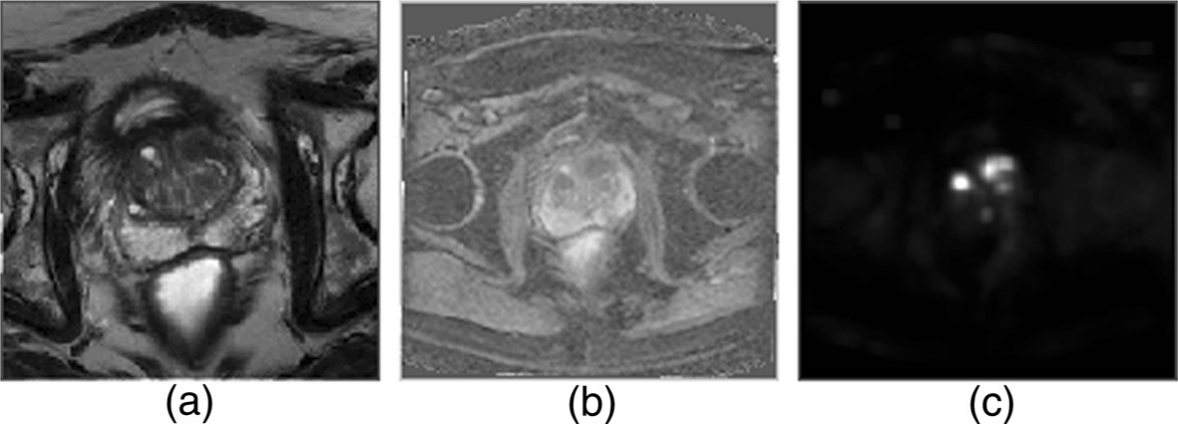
Example case 3. Tumors stand out well on CDI and poorly on T2-weighted imaging in patient with prostate cancer. a), T2-weighted imaging shows mild decrease in signal towards left side of transition zone. b), ADC map shows increased contrast around both sides of transition zone. c), CDI shows very high signal intensity corresponding to both sides of transition zone.
